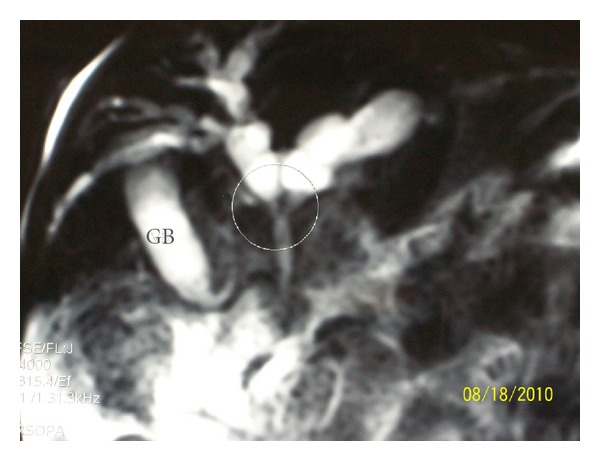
Portal cholangiopathy: this 18-year-old-boy presented with fever and jaundice. He has been treated with endoscopic sclerosis of esophageal varices from the age of six, because of portal hypertension secondary to extrahepatic portal vein obstruction. Cholangio-MRI shows dilated intrahepatic biliary tree, proximal to the level of stenosis (circle). GB: gallbladder.
